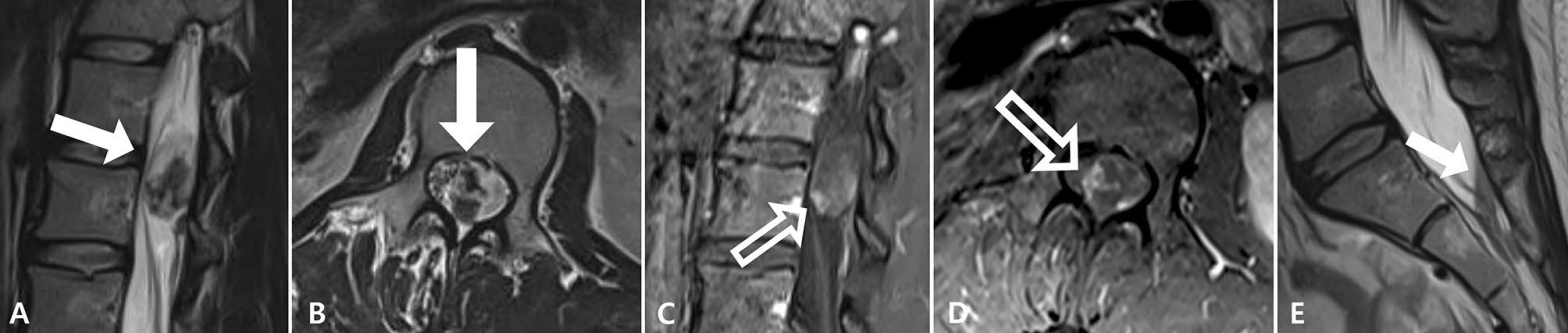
A myxopapillary ependymoma in a 25-year-old female patient at the L1-2 level. Sagittal (A) and axial (B) T2-weighted images show a 3.2 cm-sized intradural mass with heterogeneous signal intensity containing dark signal (white arrows). Sagittal (C) and axial (D) fat-suppressed contrast-enhanced T1-weighted images show focal enhancement pattern (open arrows). A fluid–fluid level is demonstrated in the distal dural sac, suggesting subarachnoid hemorrhage (E, black arrow).
